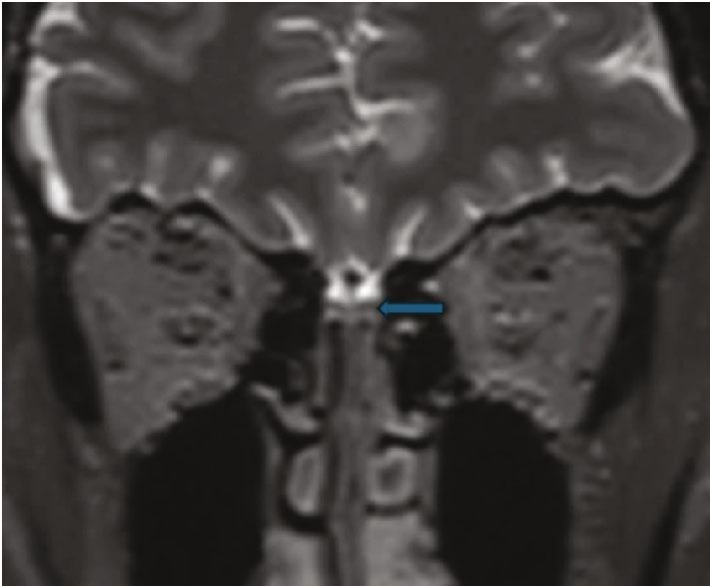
Coronal T2-weighted images (arrow) of olfactory bulb left.
